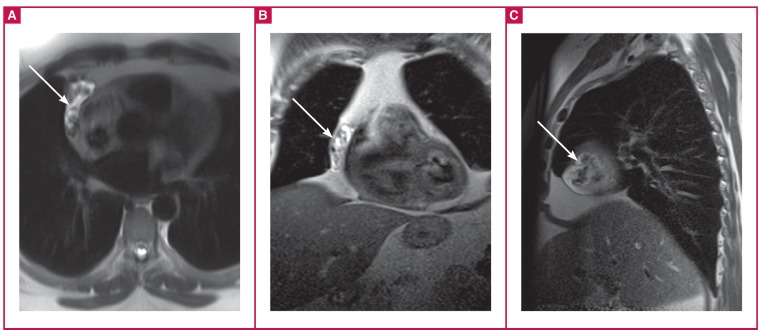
Axial (A), coronal (B) and sagittal (C) views of T2-weighted images in the paracardiac area showing a heterogeneous hyperintense mass (arrows).
